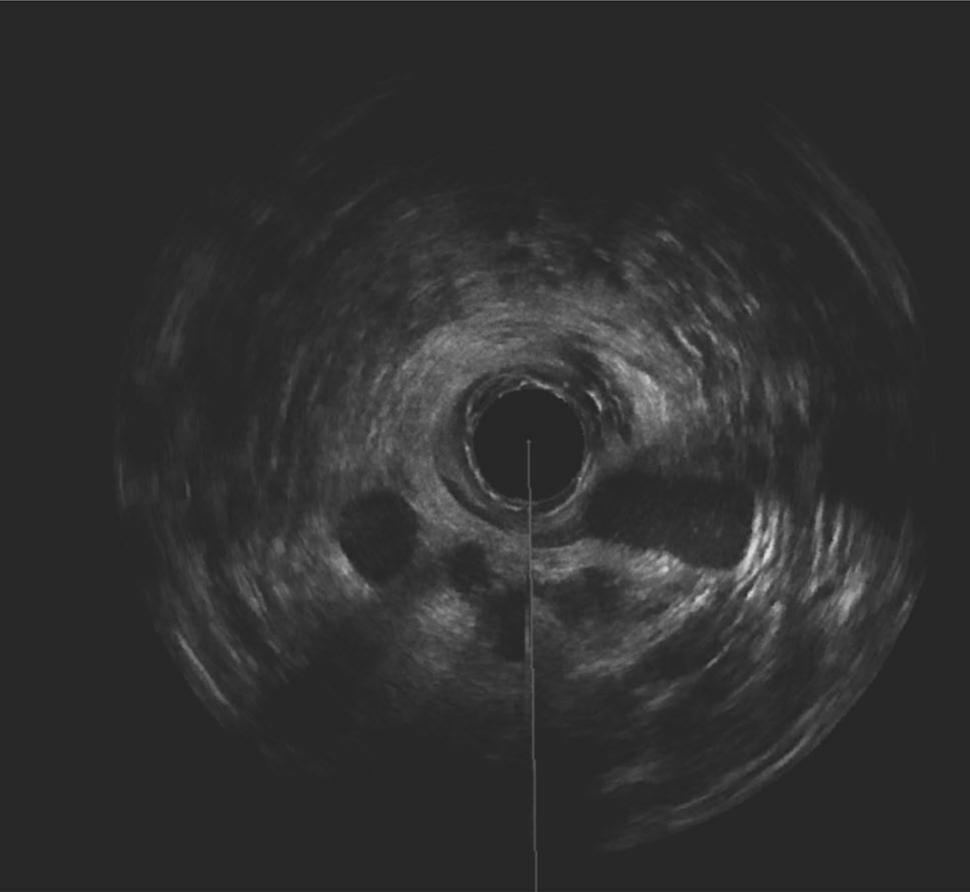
3D anal endosonography 1 month postoperatively: dislocation of 1 prosthesis at 8’o clock, in a 90° to the anal canal
